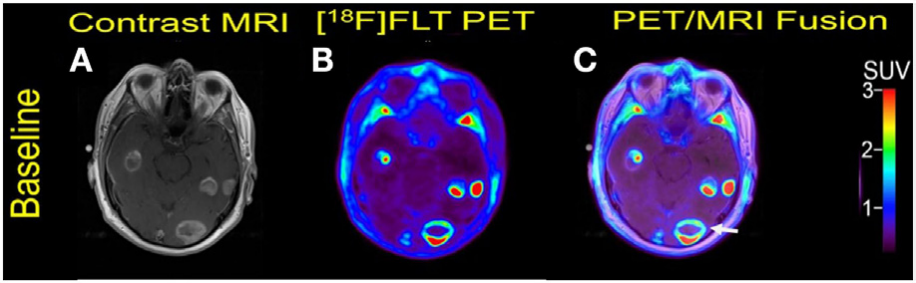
Case #5. Baseline magnetic resonance imaging (MRI) (A), fluorothymidine (FLT)-positron-emission tomography (PET) (B), and fused PET/MRI (C). Multiple FLT avid brain metastases with Gadolinium contrast enhancement were present. The largest lesion in the left occipital lobe with central necrosis (arrow) measured 2.9 cm with SUV ratio of 14.9.
